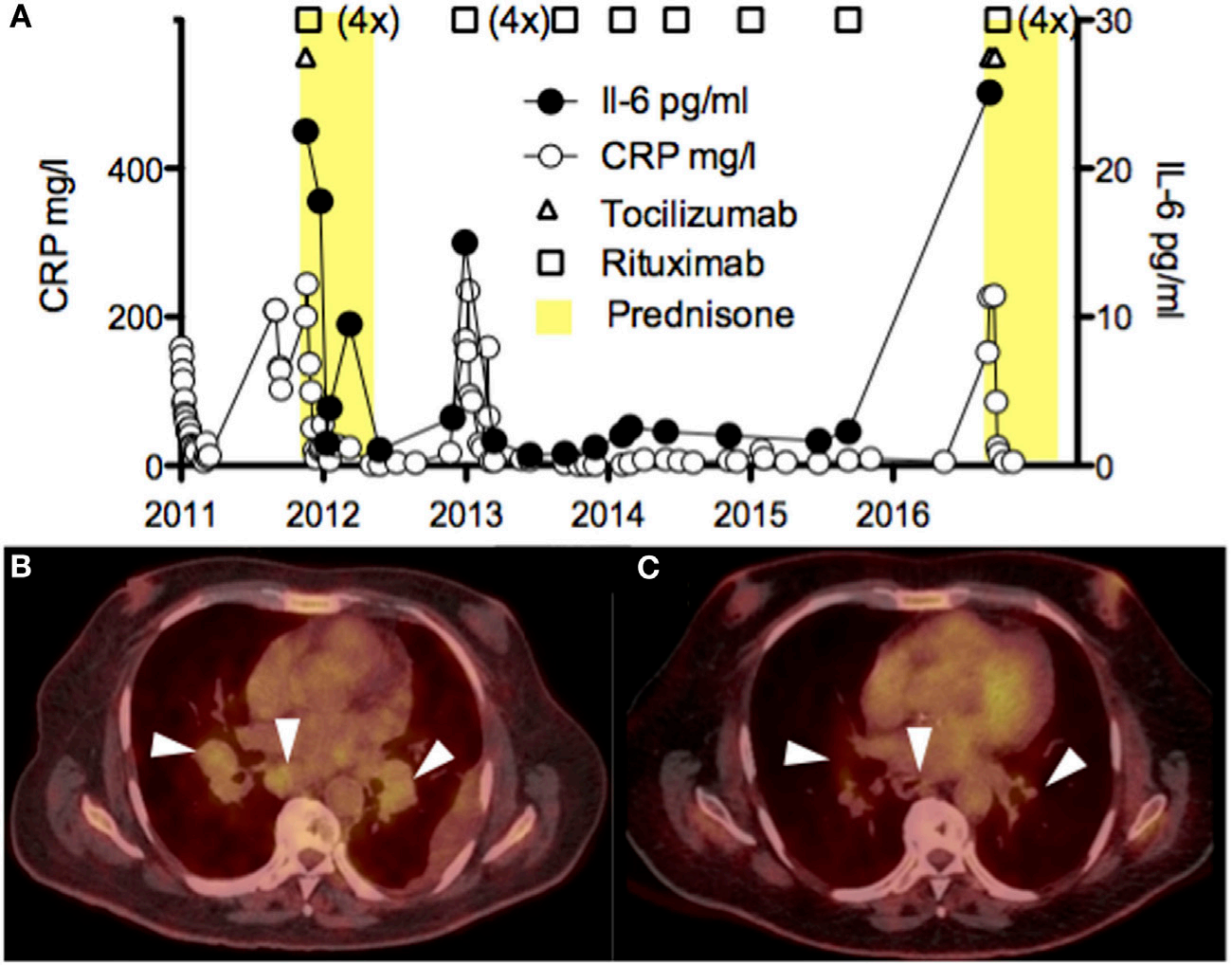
(A) Time course of the serum CRP (mg/l) and IL-6 (pg/ml) levels, and the different immunosuppressive drugs (corticosteroids, tocilizumab, and rituximab) administered to the patient. High values of IL-6 early after tocilizumab were excluded. (B,C) PET-CT scans showing pulmonary and mediastinal lymphadenopathies before (B) and after treatment with rituximab (C).
